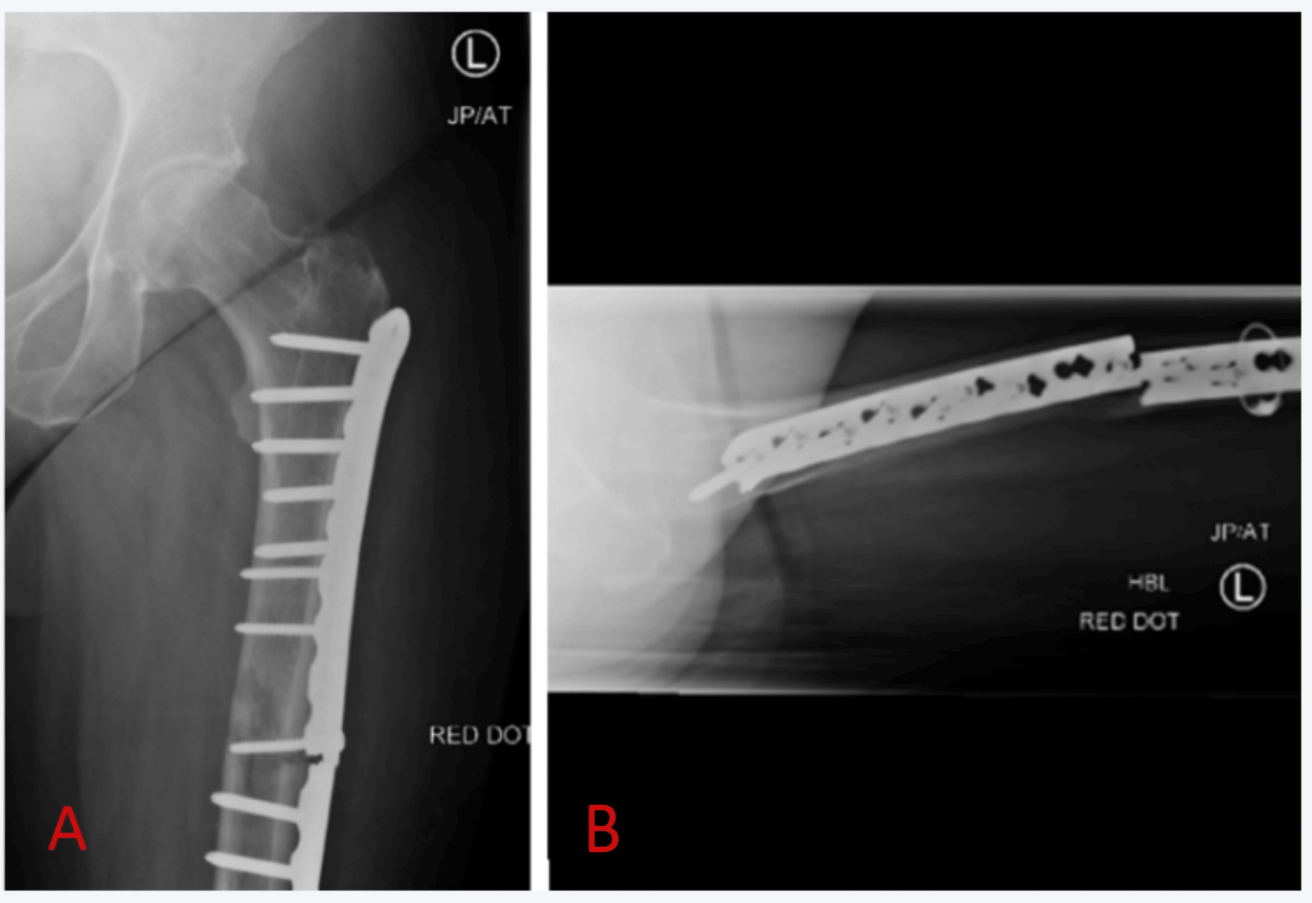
X-rays six weeks after the first revision surgery show the plate breakage at the level of the previous non-union. A: Anteroposterior X-ray of the left proximal femur; B: Axial X-ray of the left proximal femur
